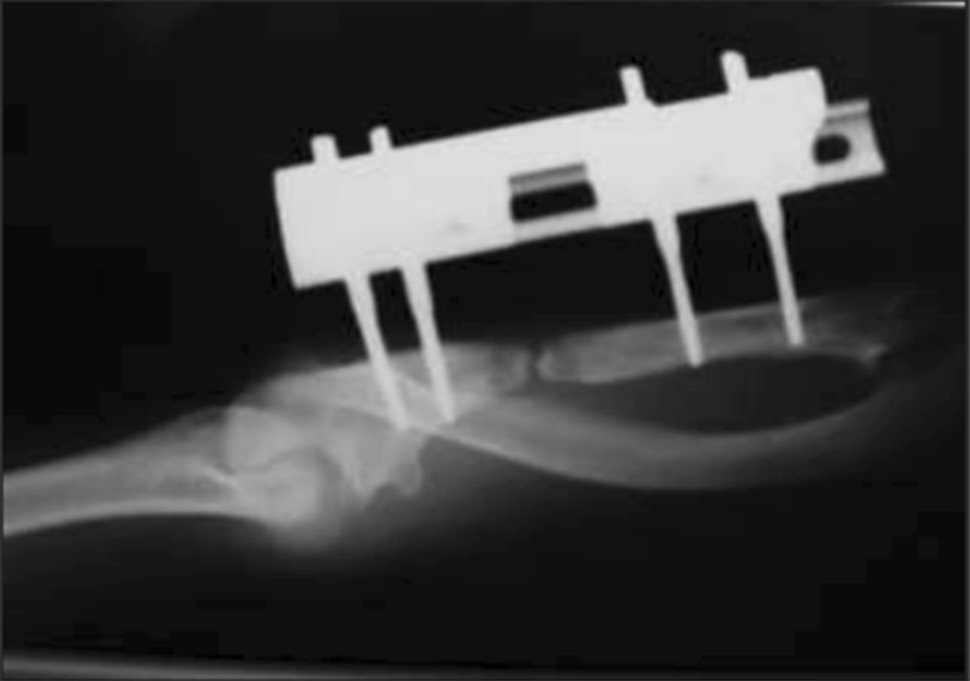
Anteroposterior radiograph of the forearm: nonunion of the ulna
